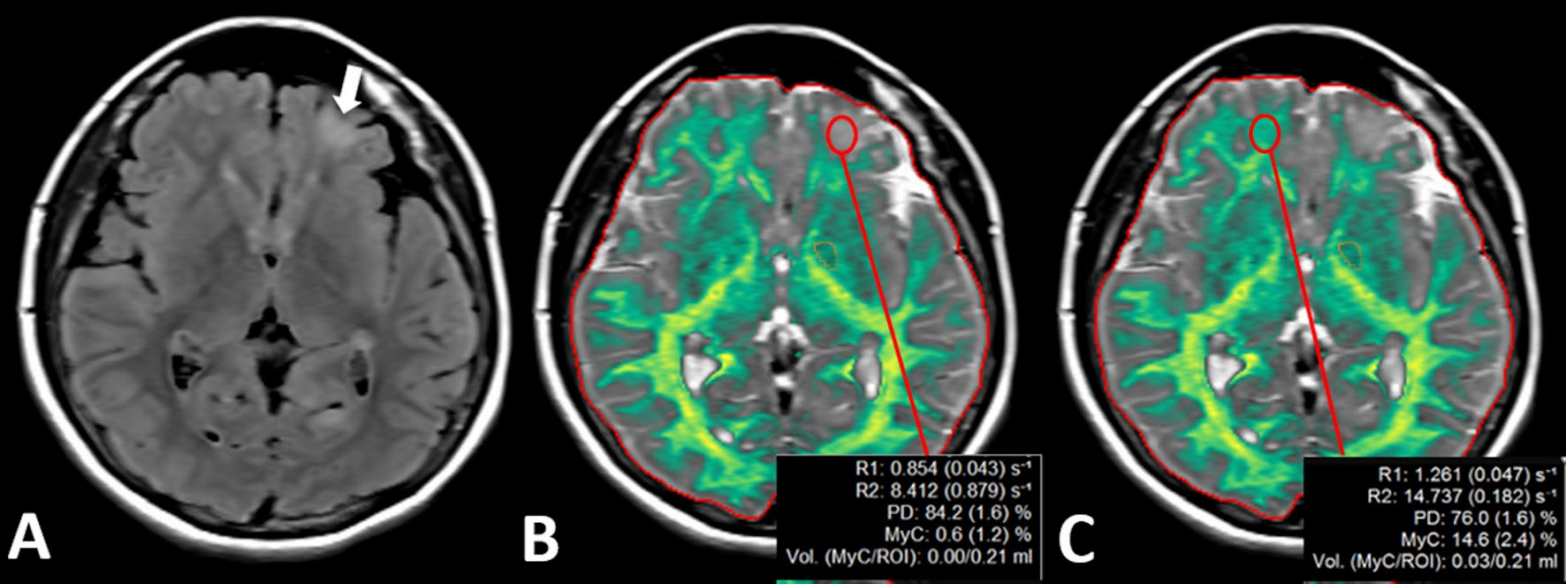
Images of 10-year-old patients with TSC. Subcortical tuber in the frontal lobe (A, white arrow) is seen on the axial FLAIR image. Myelin maps (B and C) show prominently decreased R1, R2, PD, MyC, and MyCvol values on the left side (B, red ROI) compared with the normal side (C, red ROI). Also, the myelin map does not assign the tuber as green like in other myelinated areas
